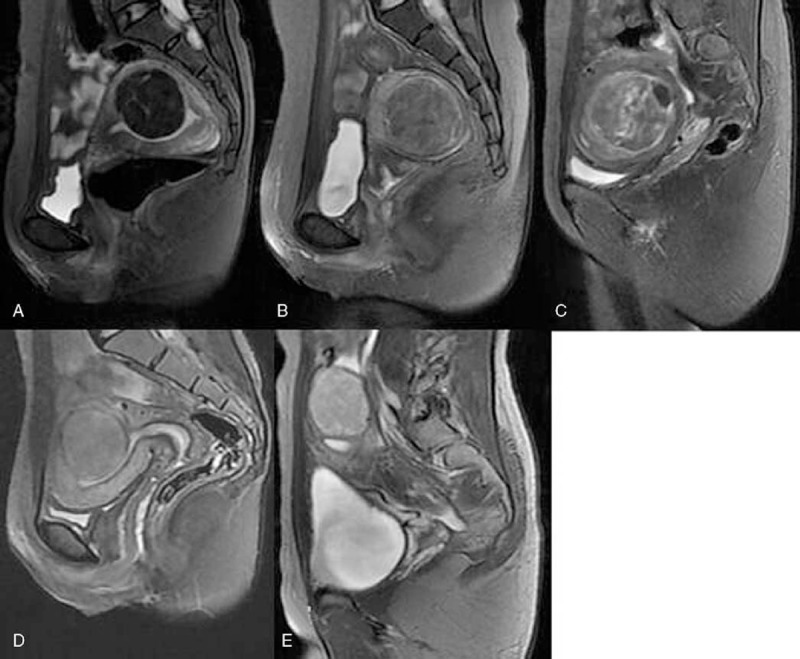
T2-weighted sagittal magnetic resonance imaging before treatment: (A) hypointense; (B) isointense; (C)–(E) hyperintense, in which (C) is heterogeneous and (D) and (E) are homogeneous; (D) slightly homogeneous hyperintense fibroid; and (E) markedly homogeneous hyperintense fibroid.
